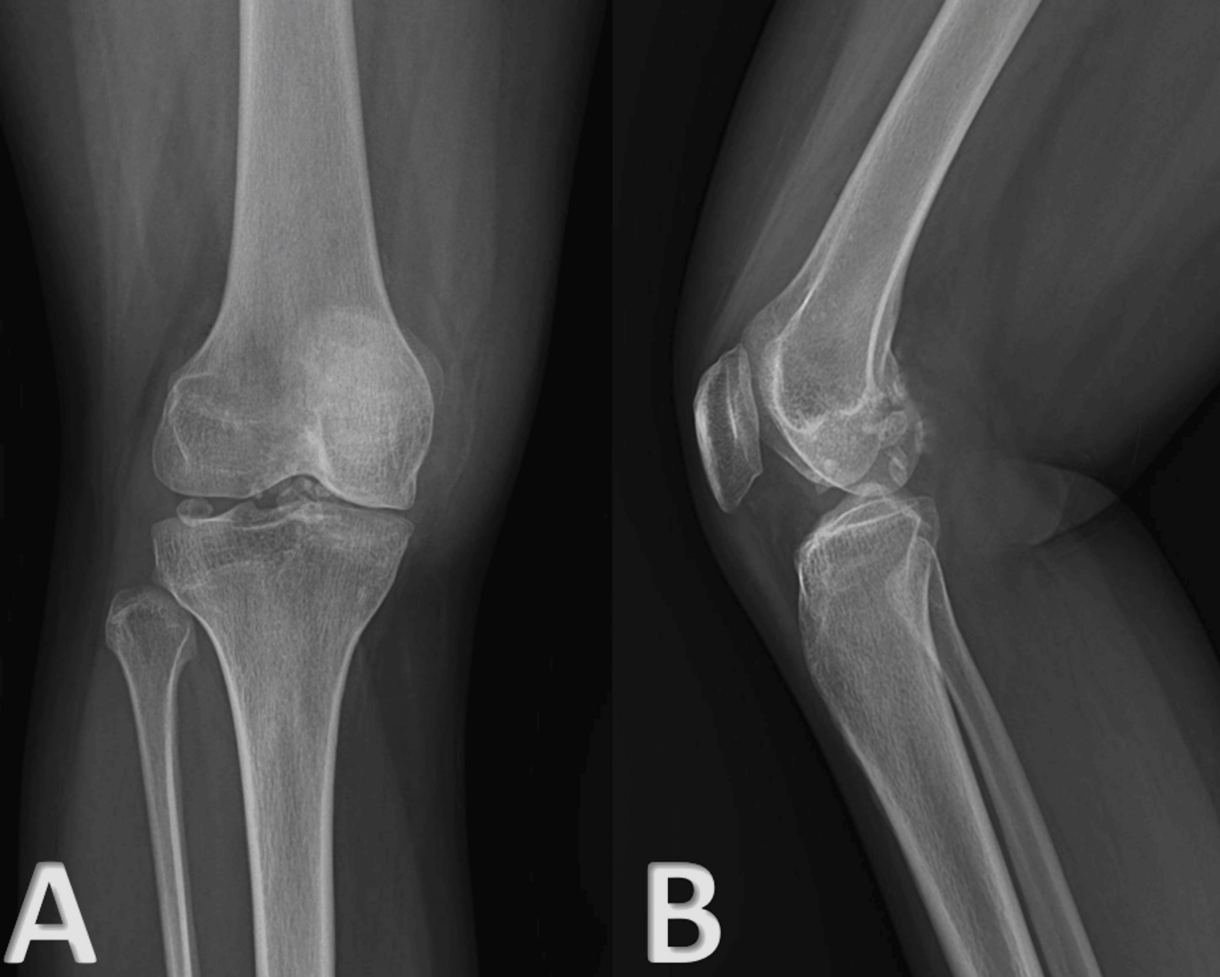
Postoperative radiographs of the left knee joint. (A) Anterior-posterior view of left knee joint. (B) Lateral view of left knee joint.
